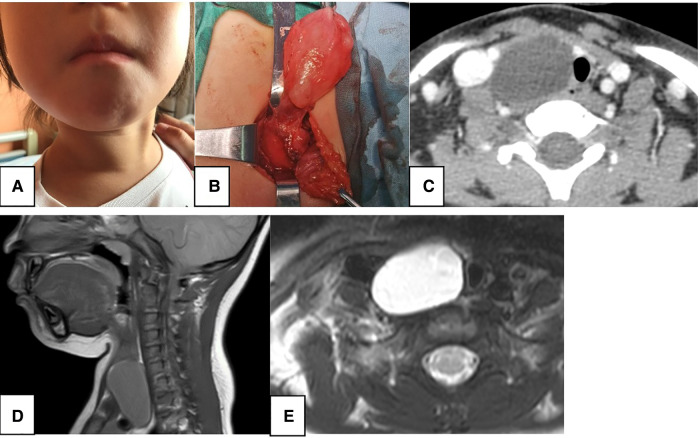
A 2.9-year-old female. CT showed a 4.2-cm sized homogeneous, low-density mass (C, white arrow) with thin walls and smooth borders in the right neck region without enhancement, and misdiagnosed as lymphangioma. The right thyroid was compressed and deformed, and the trachea (C,E, red circle) shifted to the opposite side. MRI showed low signal intensity on T1WI (D, white arrow) and high signal intensity on T2WI (E, white arrow). (A) preoperative neck mass; (B) intraoperative mass; (C) axial contrast-enhanced CT; (D) sagittal T1WI MRI; (E) axial T2WI MRI.
